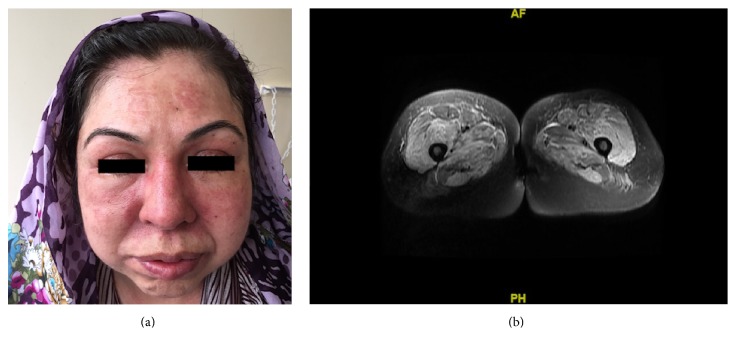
Skin and muscle involvement: (a) rash on her face and (b) bilateral diffuse inflammation in thigh muscles (magnetic resonance imaging).
